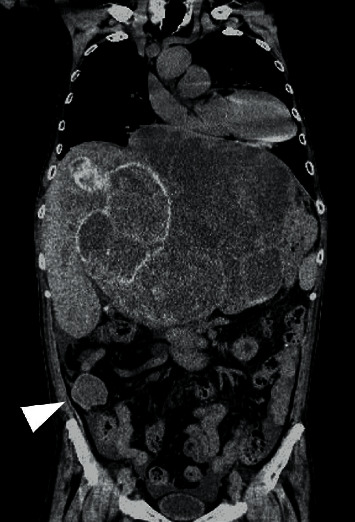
CT findings one month before death. Multiple metastases of GIST are found in the liver. A 40 mm nodule is found in the ileocecal region (arrowhead). No tumor was found in both lungs.
